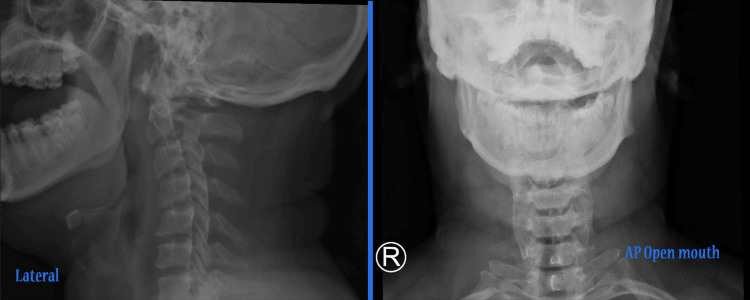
Anterior/posterior open mouth and lateral radiograph follow-up after six weeks of injury.
